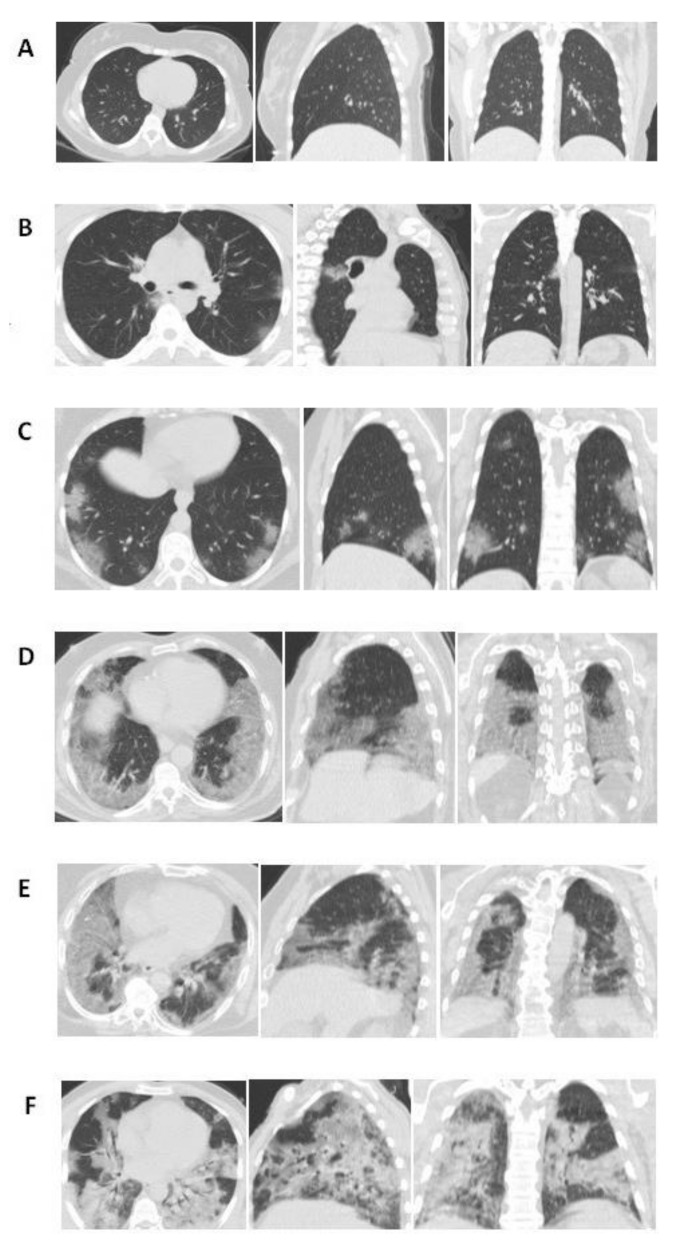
Chest CT findings of COVID-19 showthe CT score of right lower lobe involvement on axial, sagittal, and coronal images. (A) Score 0, 0% involvement; (B) Score 1, <5% involvement; (C) Score 2, 5–25% involvement; (D) Score 3, 26–49% involvement; (E) Score 4, 50–75% involvement; (F) Score 5, >75% involvement. CT: Computed tomography.
